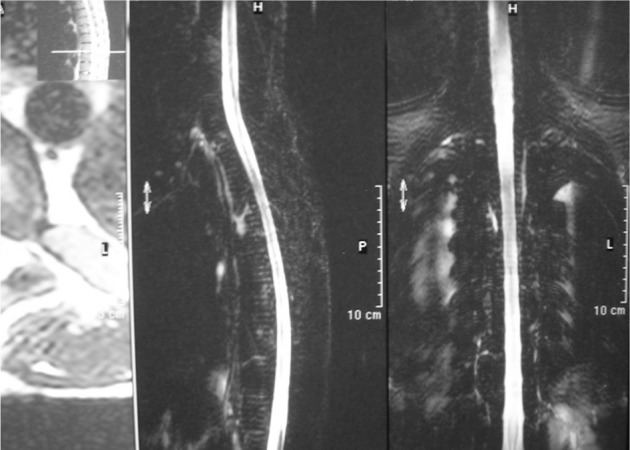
After transfusion, MRI showed complete resolution of the extramedullary mass.
